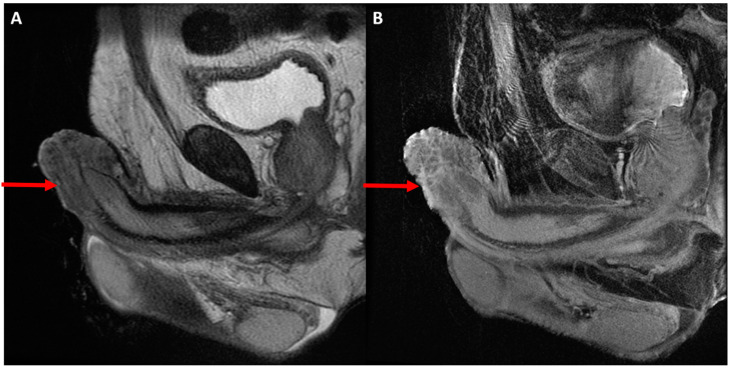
51-year-old male with newly diagnosed penile cancer of the glans penis presents for MRI to evaluate the depth and degree of local invasion and for surgical planning. Sagittal T2-weighted (A) and T1 fat suppressed postcontrast (B) images demonstrate the known mass at the glans penis with intermediate T2 signal intensity and hypoenhancement invading into the adjacent corpora. Of note, the degree of corporal invasion is more clearly demonstrated on postcontrast images in this case.
